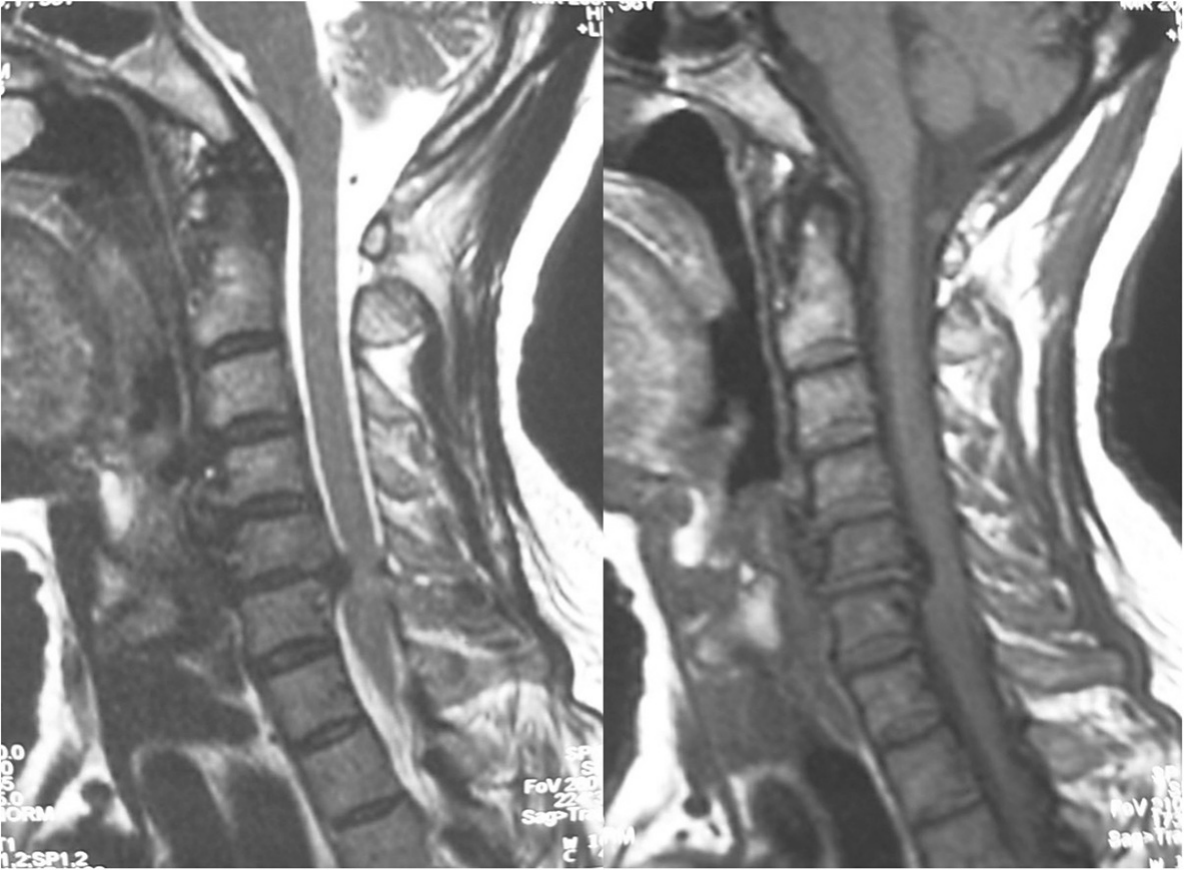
T1-weighted (left) and T2-weighted (right) images demonstrate disk degeneration without Modic endplate change (Modic-0).
